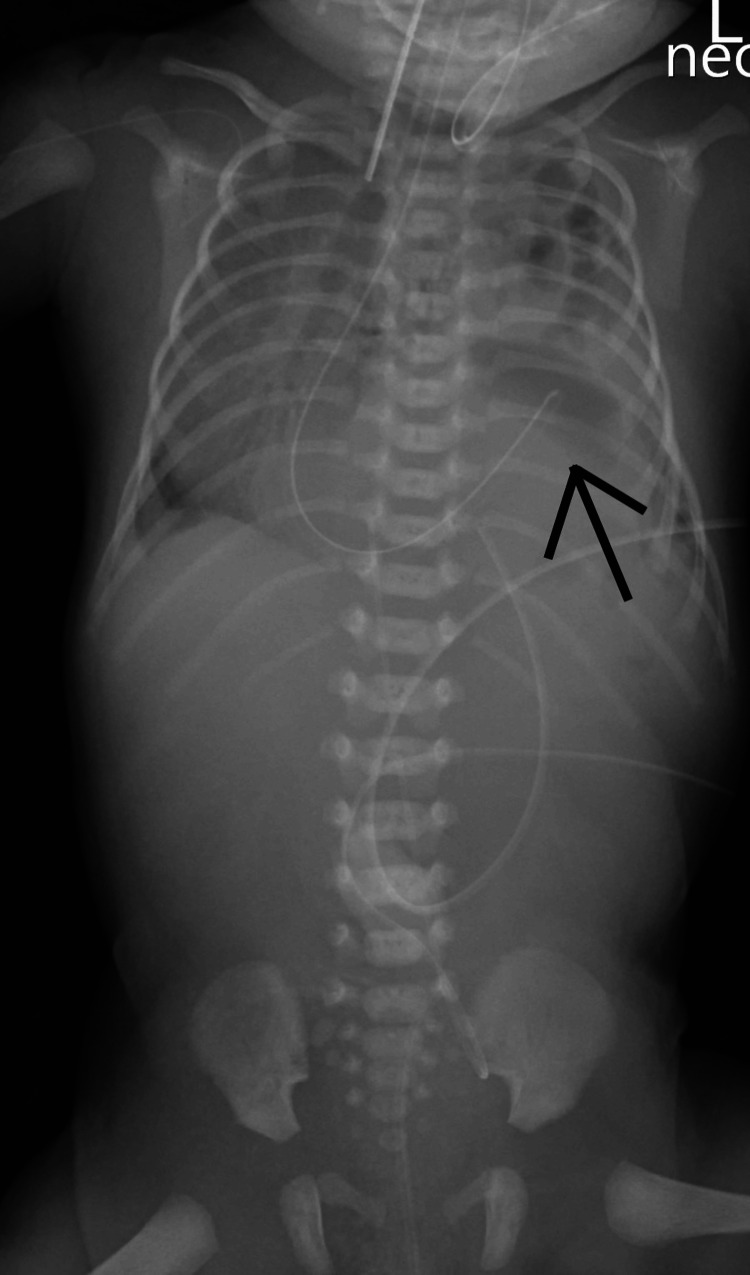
The X-ray before diaphragmatic reconstruction The arrow points at the herniated bowel loops and stomach within the left hemithorax. Additionally, the chest X-ray shows a mediastinal shift.
